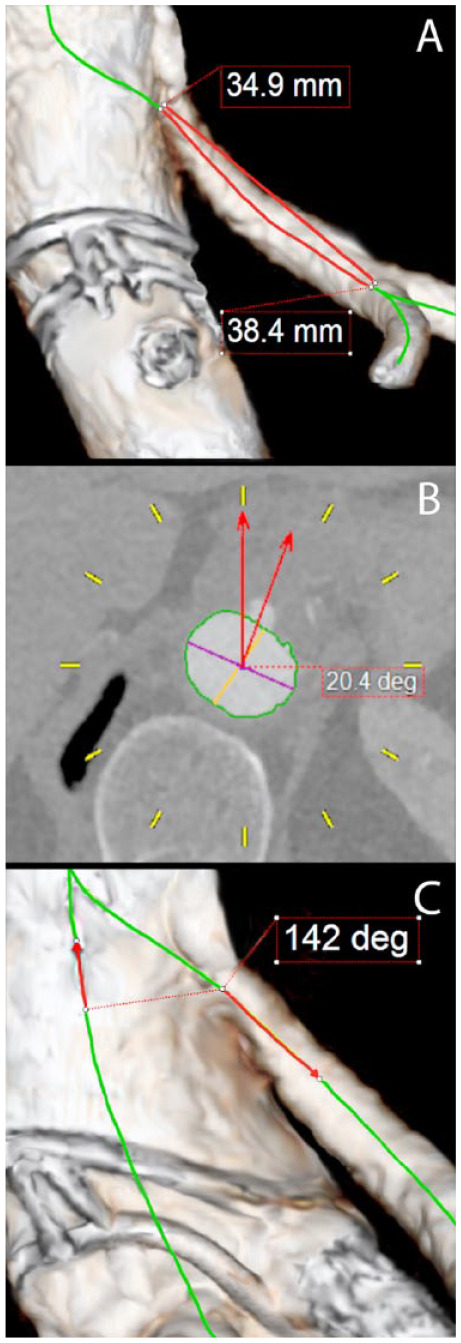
Reconstructions of the aorta from computed tomography angiography
